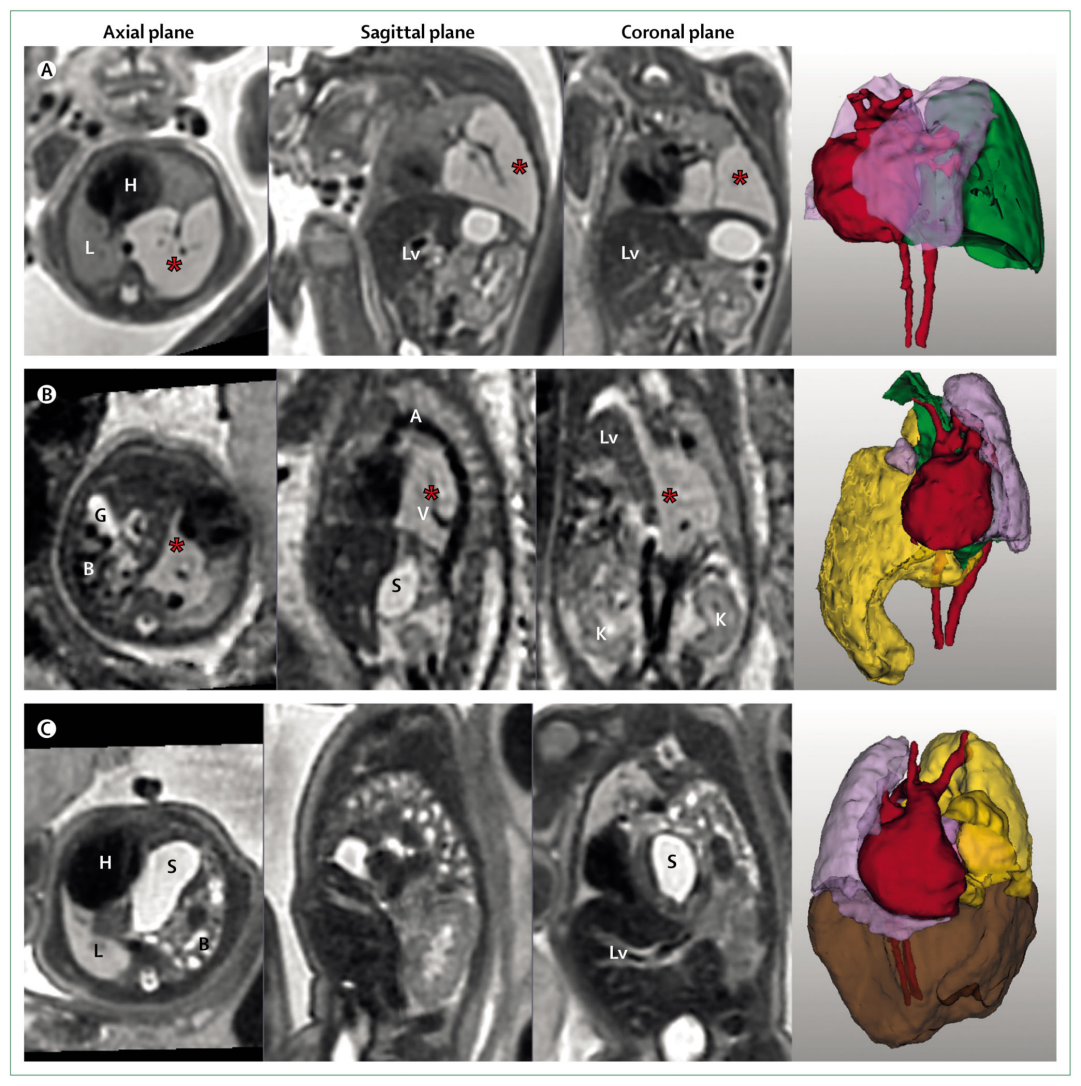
T2-weighted MRI and three-dimensional models of three fetuses with lesions of the thorax (A) Fetus of 24 weeks’ gestation with a lesion of left lower lung showing a microcystic congenital pulmonary airway malformation of the left lower lobe (marked by an asterisk), also shown in 3D (green), with heart (red) and lungs (lilac). (B) Fetus of 24 weeks’ gestation with bronchopulmonary sequestration (marked by an asterisk) and right congenital diaphragmatic hernia. Reorientation to true sagittal shows a feeding vessel arising from the aorta. Note the right-sided diaphragmatic hernia containing the bowel (yellow), gallbladder, and stomach. Kidneys are shown in the coronal plane. 3D models show bronchopulmonary sequestration (green), lungs (lilac), and heart (red). (C) Fetus of 33 weeks’ gestation with a left-sided congenital diaphragmatic hernia, containing stomach and intestine (yellow), and liver (brown) within the hernia, and showing the heart (red) and lung (lilac) in relation to hernial content. 3D=three-dimensional. A=aorta. B=bowel. G=gallbladder. H=heart. K=kidney. L=lung. Lv=Liver. S=stomach. V=vessel.
